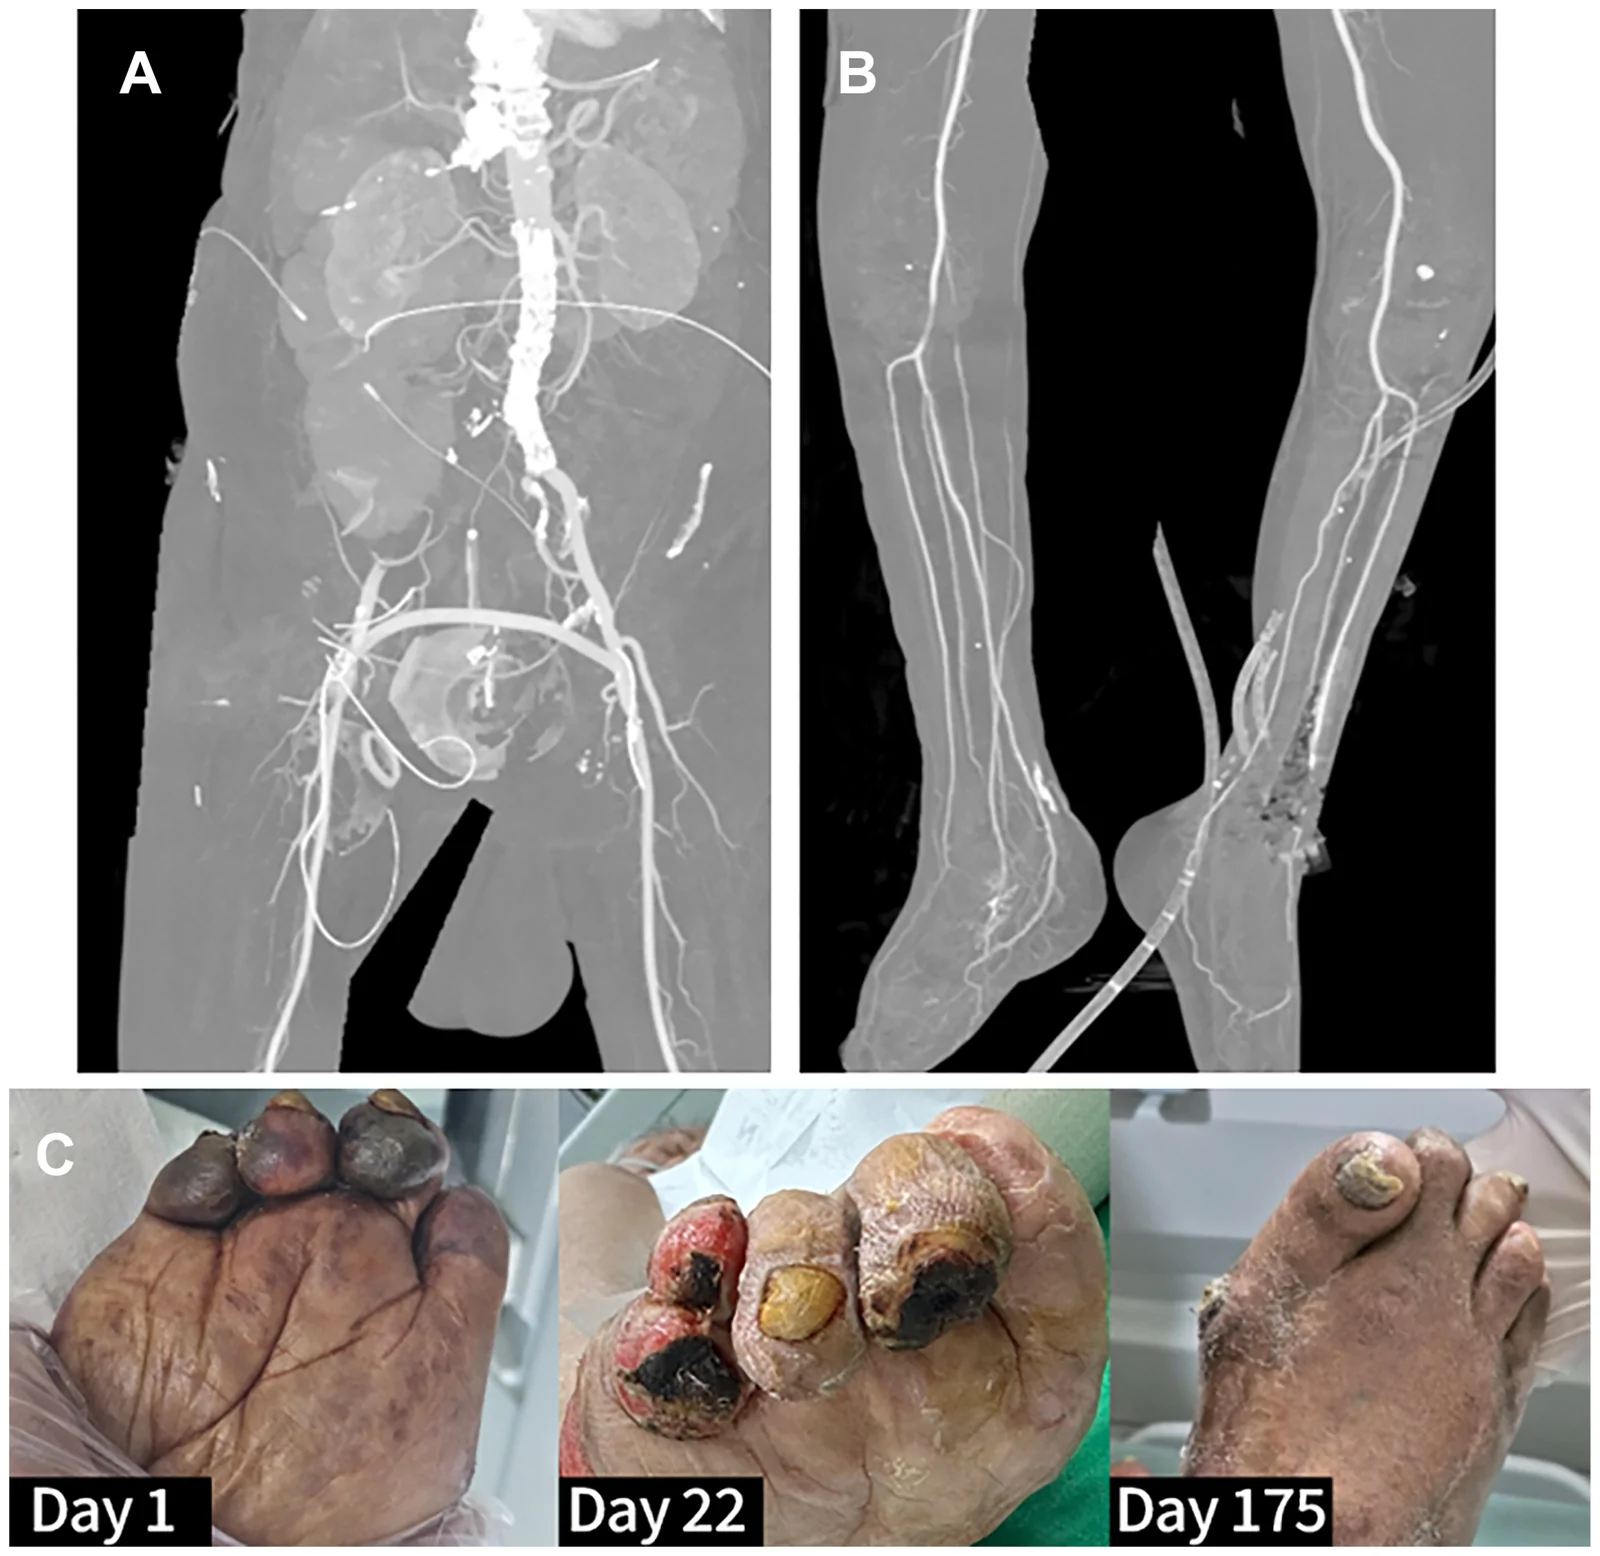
Postoperative outcomes. A, Postoperative computed tomography angiography demonstrating successful exclusion of the aneurysm and iliocaval fistula. B, Maximum intensity projection image demonstrating restoration of arterial inflow with normalization of arterial caliber in the right lower extremity. C, Serial photographs of the right foot demonstrating progressive wound healing from admission through outpatient follow-up. Labels indicate days after admission.
